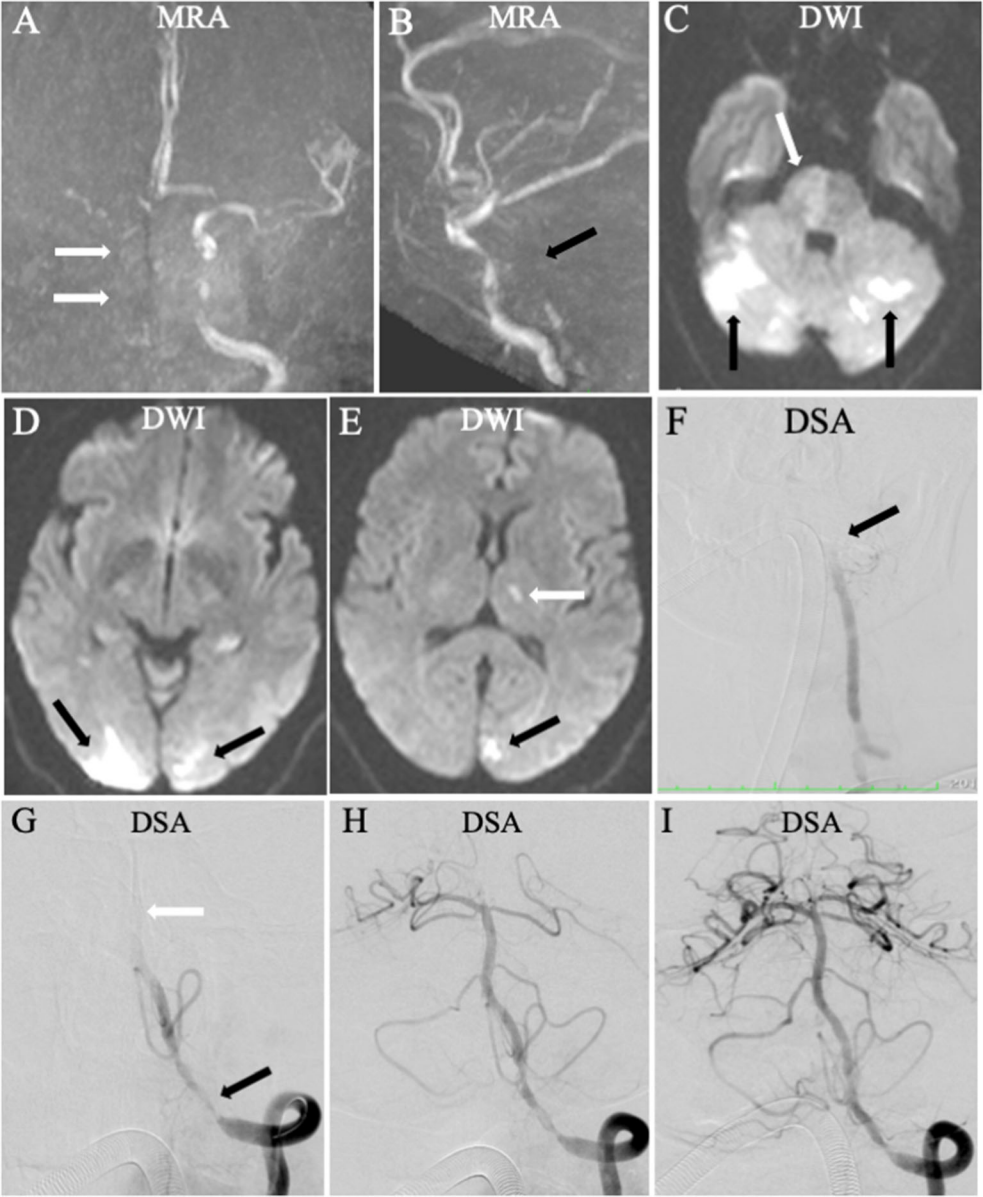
Endovascular treatment (EVT) for a patient with higher National Institutes of Health Stroke Scale (NIHSS) score: 20 and lower Acute Stroke Prognosis Early Computed Tomography Score (ASPECTS). Magnetic resonance angiography displayed the right internal carotid artery occlusion [(A), arrow] and the vertebrobasilar artery occlusion [(B), black arrow]. Diffusion-weighted imaging showed multiple acute ischemic stroke in posterior circulation involving the right pons [(C), white arrow], bilateral cerebellum [(C), black arrow], bilateral occipital lobe [(D), black arrow], and left ganglion [(E), white arrow]. The Acute Stroke Prognosis Early Computed Tomography Score (ASPECTS) and pons–midbrain index were 3 and 2, respectively. Based on the findings of DWI and MRA, the left vertebral and basilar artery was diagnosed as the “criminal” artery. Digital subtraction angiography (DSA) confirmed the artery occlusion for the left vertebral and basilar artery [(F, G), white arrow). Endovascular treatment was performed with a stent retriever (H), resulting in successful reperfusion with the modified thrombolysis in cerebral infarction 2b (I). The modified Rankin Scale (mRS) score was 3 at 90 days.
